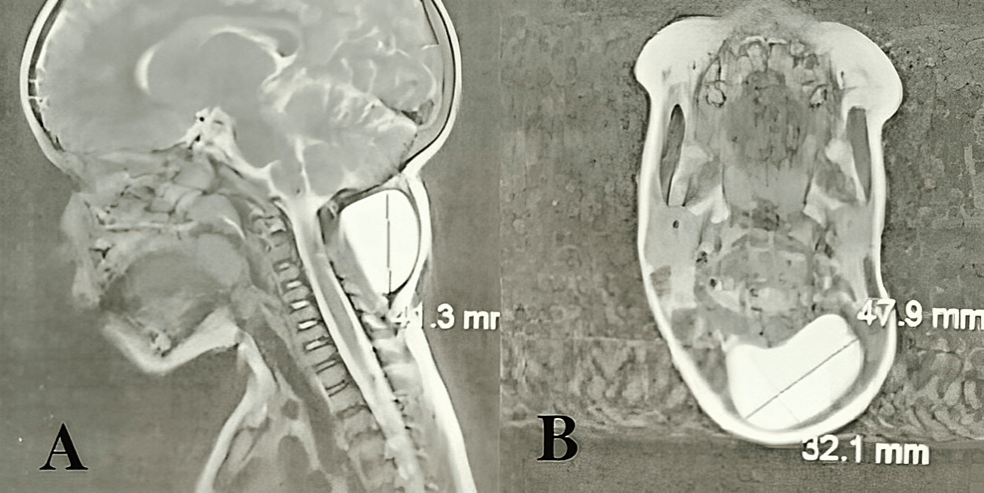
Cervical MRI of the neck cyst A: Cervical MRI in T2-weighted sequence sagittal plane showing a well-demarcated cystic lesion posterior to the vertebrae, extending from C1 to C5 with no apparent invasion of the adjacent tissue. B: Cervical MRI in T2-weighted sequence axial plane showing the same well-demarcated cystic lesion.
